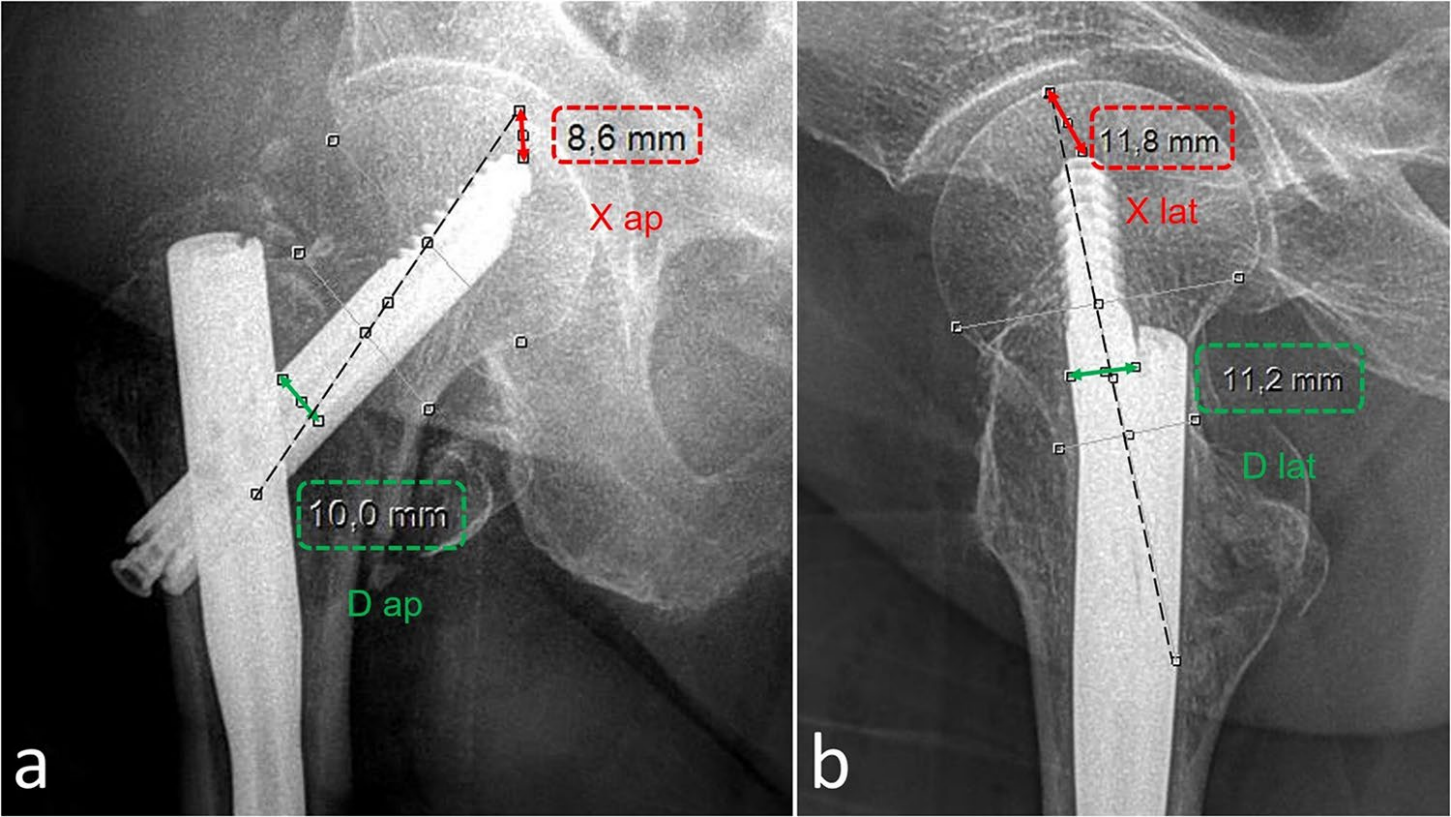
Measurement and calculation of the Tip-Apex Distance. TAD = (X-ap x D-true/D-ap) + (X-lat x D-true/D-lat). Measurements on AP radiograph (a). Measurements on lateral radiograph (b)
